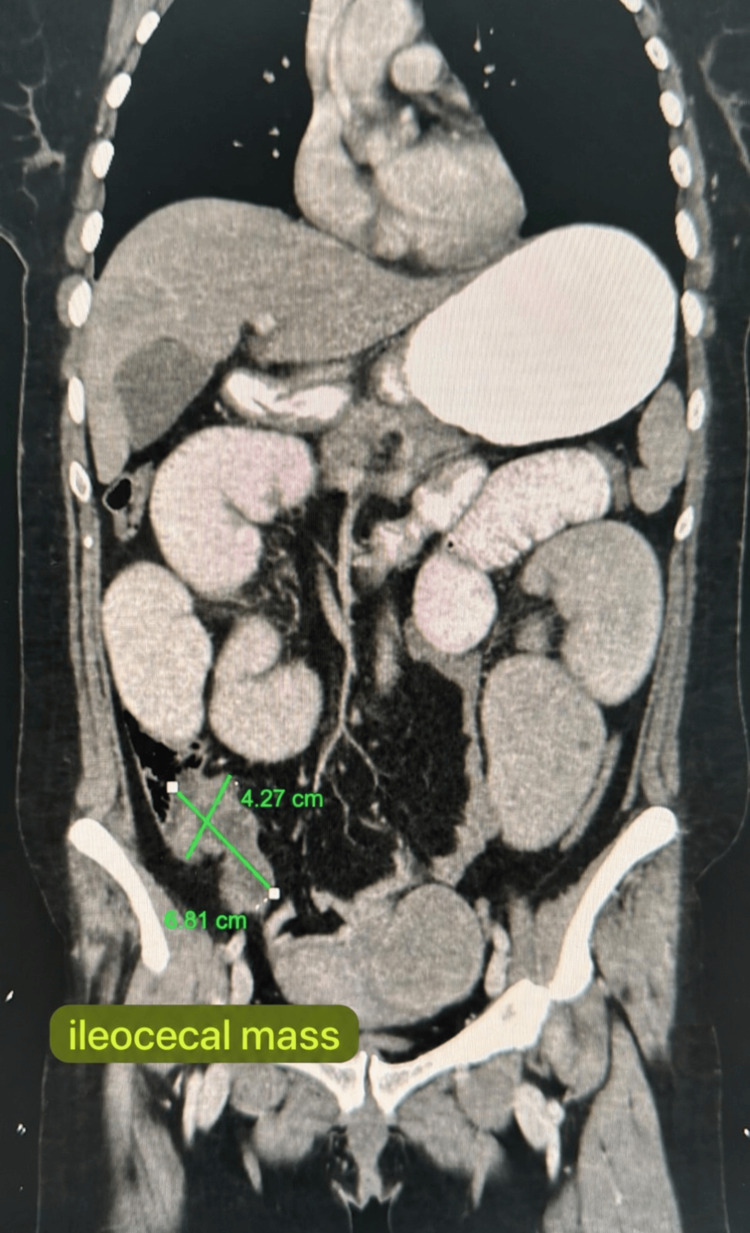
A CT scan of the abdomen demonstrating an ileocecal mass
